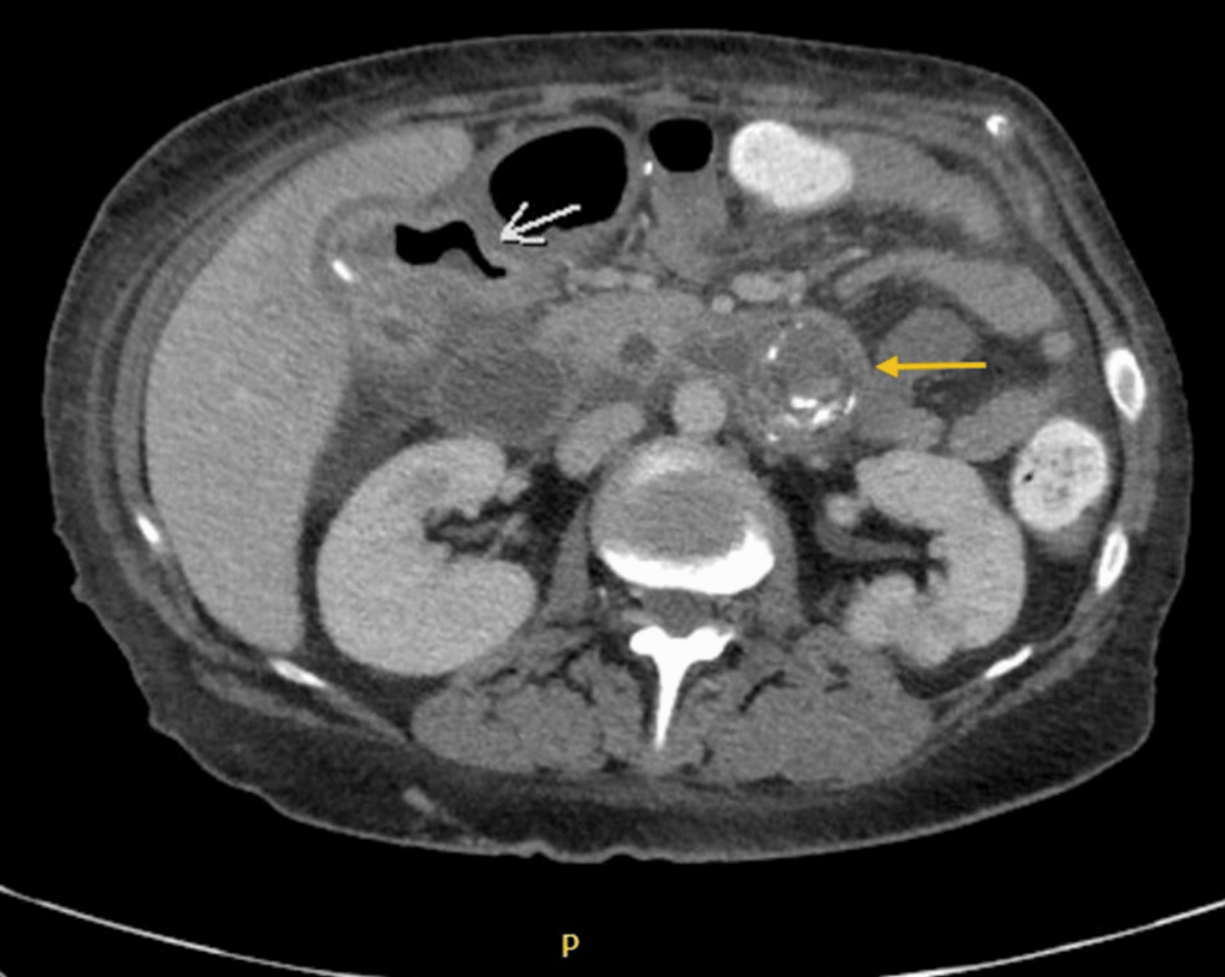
Contrast-enhanced axial CT image of cholecystoduodenal fistula (white arrow) and large jejunal gallstone (gold arrow).
